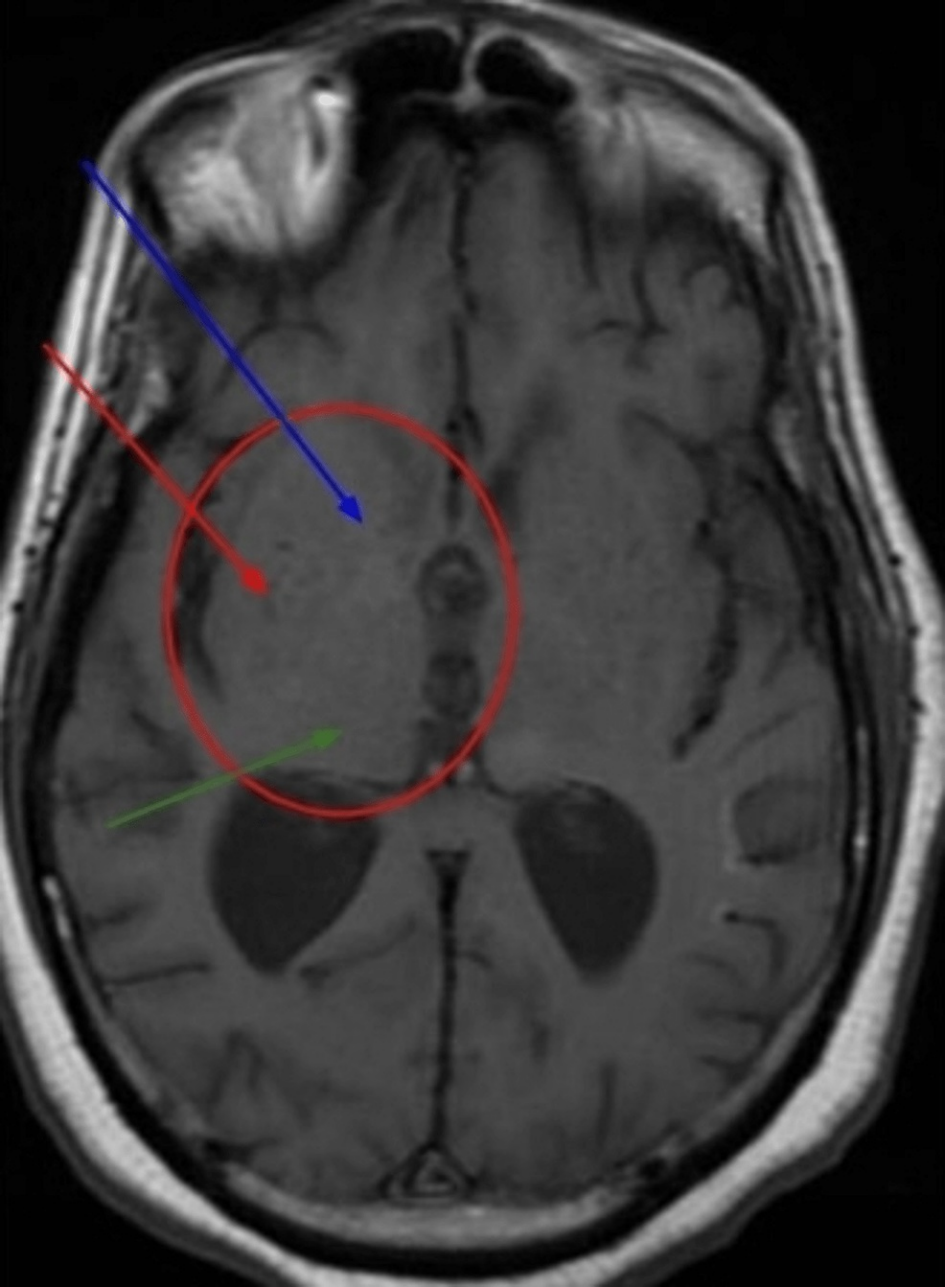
Brain MRI, T1 Bilateral basal ganglia atrophy (caudate, lentiform, and thalamus). Blue arrow: caudate, Red: lentiform, Green: thalamus, Circle: basal ganglia atrophy.
